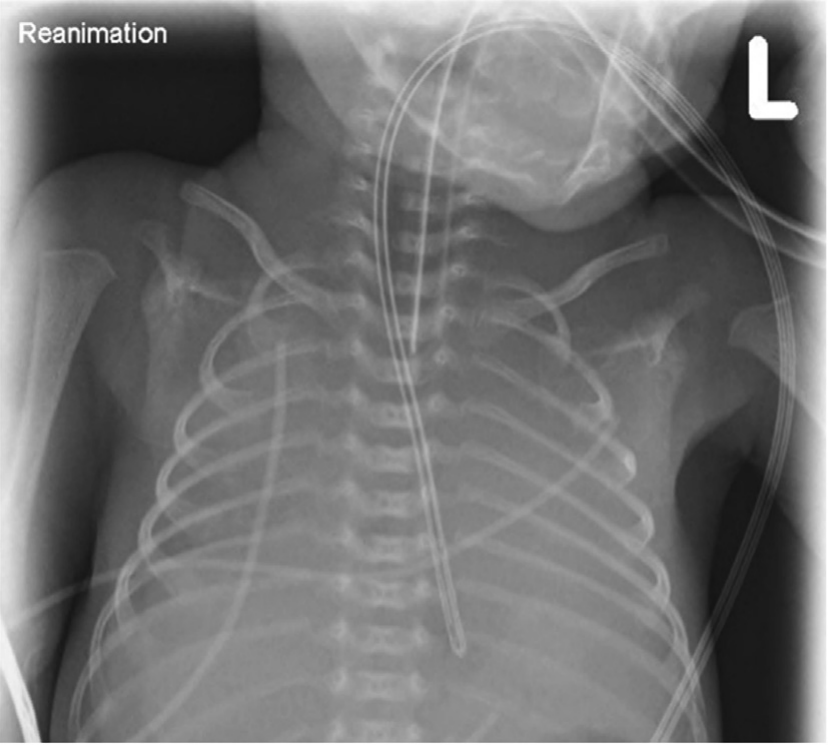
Chest x-ray during cardiopulmonary resuscitation, 18 min after birth.
